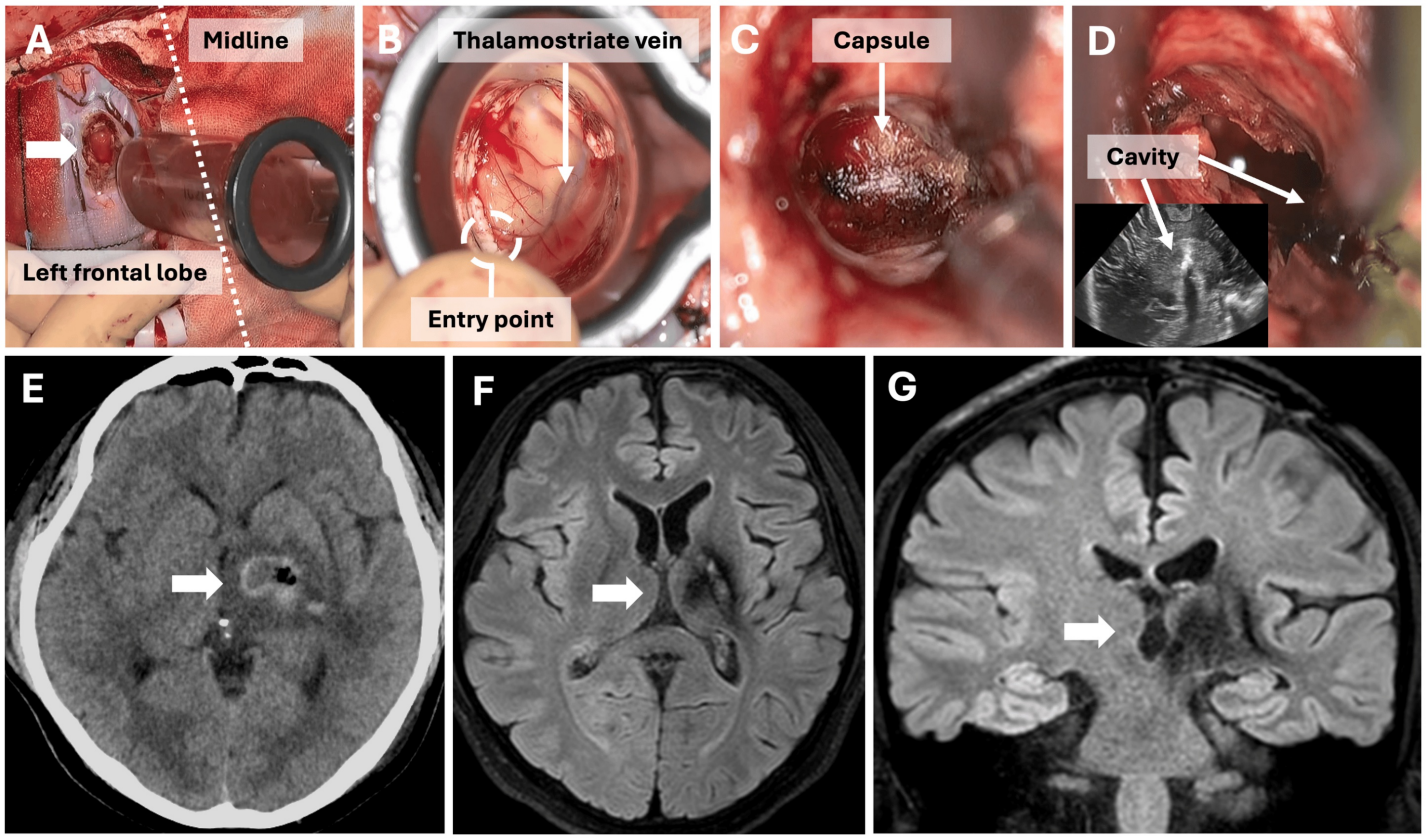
Intraoperative and postoperative images (Case 1) A 1 × 2 cm corticotomy was performed 9 cm parietal and 1.5 cm lateral to the nasion (A, arrow). The ViewSite Brain Access System (21 mm × 7 cm) was inserted into the left lateral ventricle along with the corticotomy, and the entry point into the hemorrhage was determined to be 1 cm lateral to the thalamostriate vein, which was the closest point to the hemorrhage (B). The hematoma capsule and vascular conglomerates were dissected (C arrow). Subtotal removal of the hematoma capsule was confirmed using intraoperative ultrasonography (D arrows). Computed tomography on postoperative day 1 shows a reduction in hematoma with no further bleeding or infarction (E, arrow). Magnetic resonance imaging at the six-month follow-up showing further shrinkage of the residual lesion (F and G, arrows).
